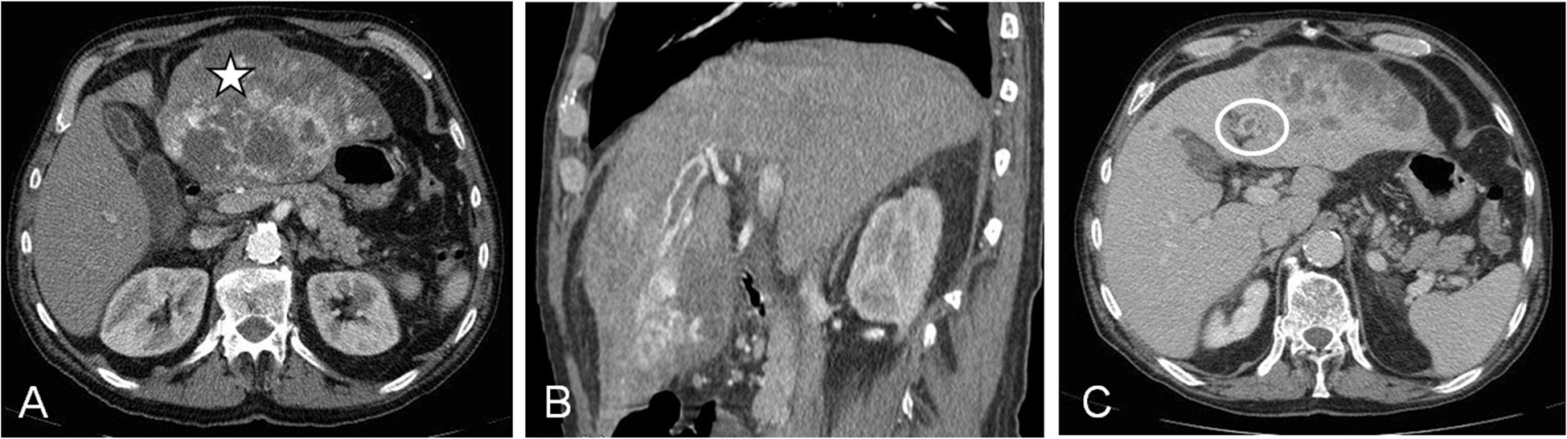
Computerized tomography (CT) scan features of the liver tumor. Liver tumor with (A) arterial enhancement (star), (B) portal washout and (C) portal vein thrombosis (PVT) (circle). CT, computerized tomography; PVT, portal vein thrombosis.
